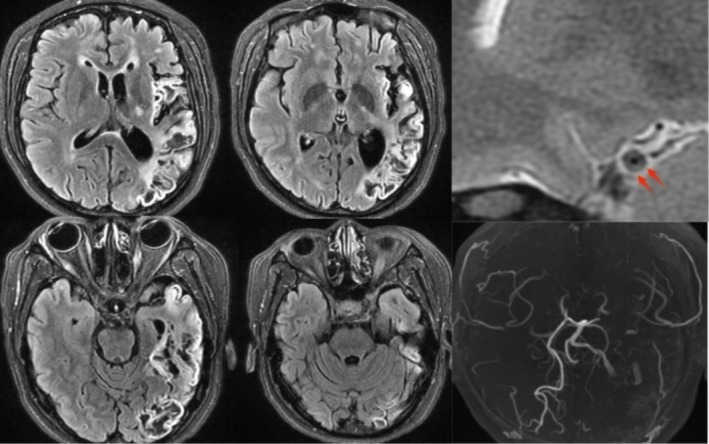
Fifty‐two‐year‐old male patient with MMD presented with imaging findings including: Left MCA territory infarction on FLAIR sequence. HRMRI showed concentric wall thickening and negative remodeling of the left middle cerebral artery (MCA) (red arrow). TOF‐MRA revealed signal loss in bilateral MCAs, bilateral anterior cerebral arteries and the terminal left internal carotid artery, along with signal discontinuity at the left posterior cerebral arteries (PCA) P2 segment, while the right PCA showed good visualization with compensatory dilatation.
